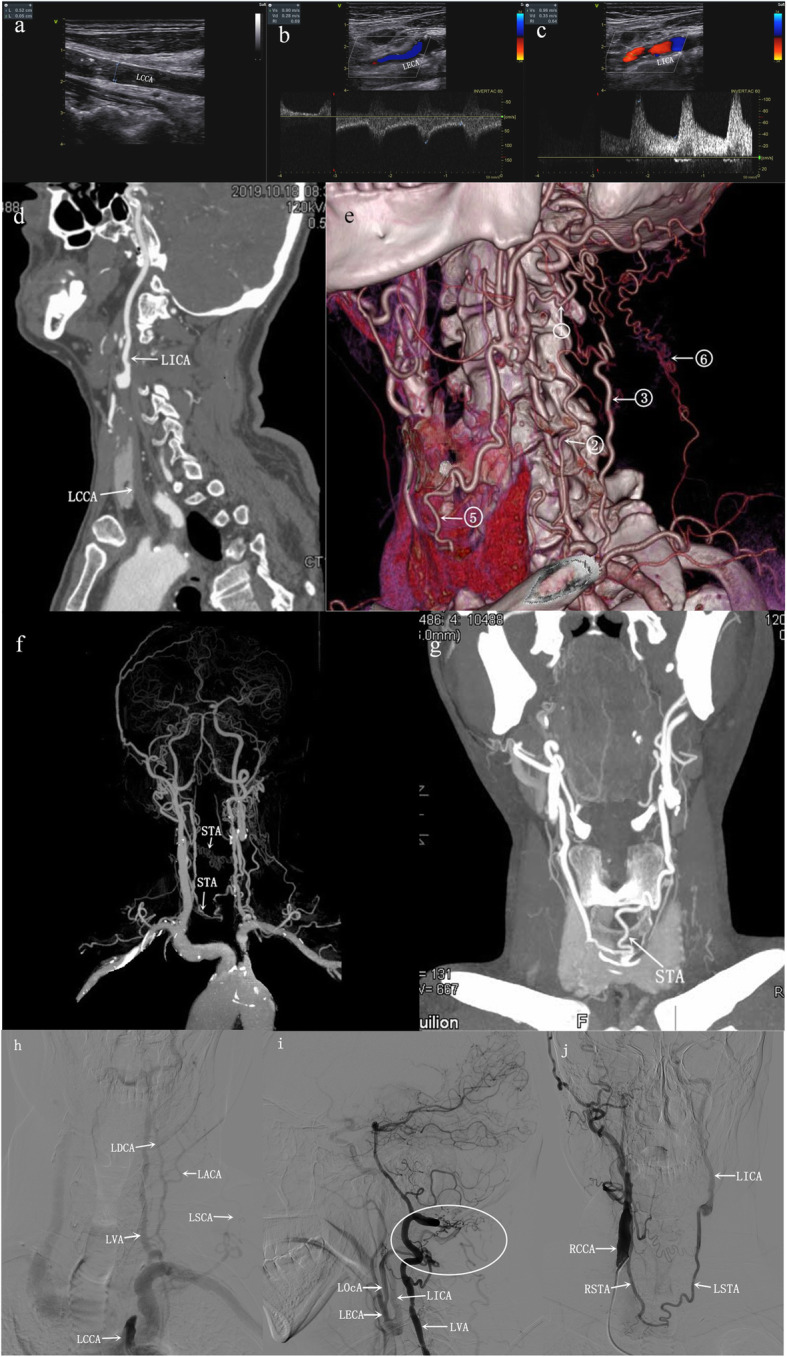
Patient 4, male. (Fig. 3a-c) Vascular ultrasound shows: a The left CCA had no blood flow, b the left ECA had retrograde blood flow, c left ICA blood flow shows low fluctuations in blood flow spectrum changes, (Fig. 3d-g) CTA shows: d CPR shows: The left CCA occlusion, left ICA patency, e VR shows: Internal carotid artery stealing pathway, f VR and g MIP shows: contralateral superior thyroid artery - affected superior thyroid artery forms an anastomotic blood vessel. (Fig. 3h-j) DSA showed: h Left superficial cervical artery positive image: the left CCA occlusion, the left ascending cervical artery (ACA), the deep cervical artery (DCA) and the superficial cervical artery (SCA) were thickened and lengthened. i Left vertebral artery lateral images display stealing pathway: left vertebral artery (VA), left ascending carotid artery (ACA), left deep carotid artery, left superficial carotid artery - left occipital artery (OcA) - left external carotid artery - left internal carotid artery . The circular area indicates that vertebral artery, deep carotid artery, ascending carotid artery and superficial carotid artery form anastomotic vessels. j Right common carotid artery anteroposterior images display stealing pathway: right common carotid artery - right superior thyroid artery (STA) - left superior thyroid artery - left external carotid artery - left internal carotid artery
